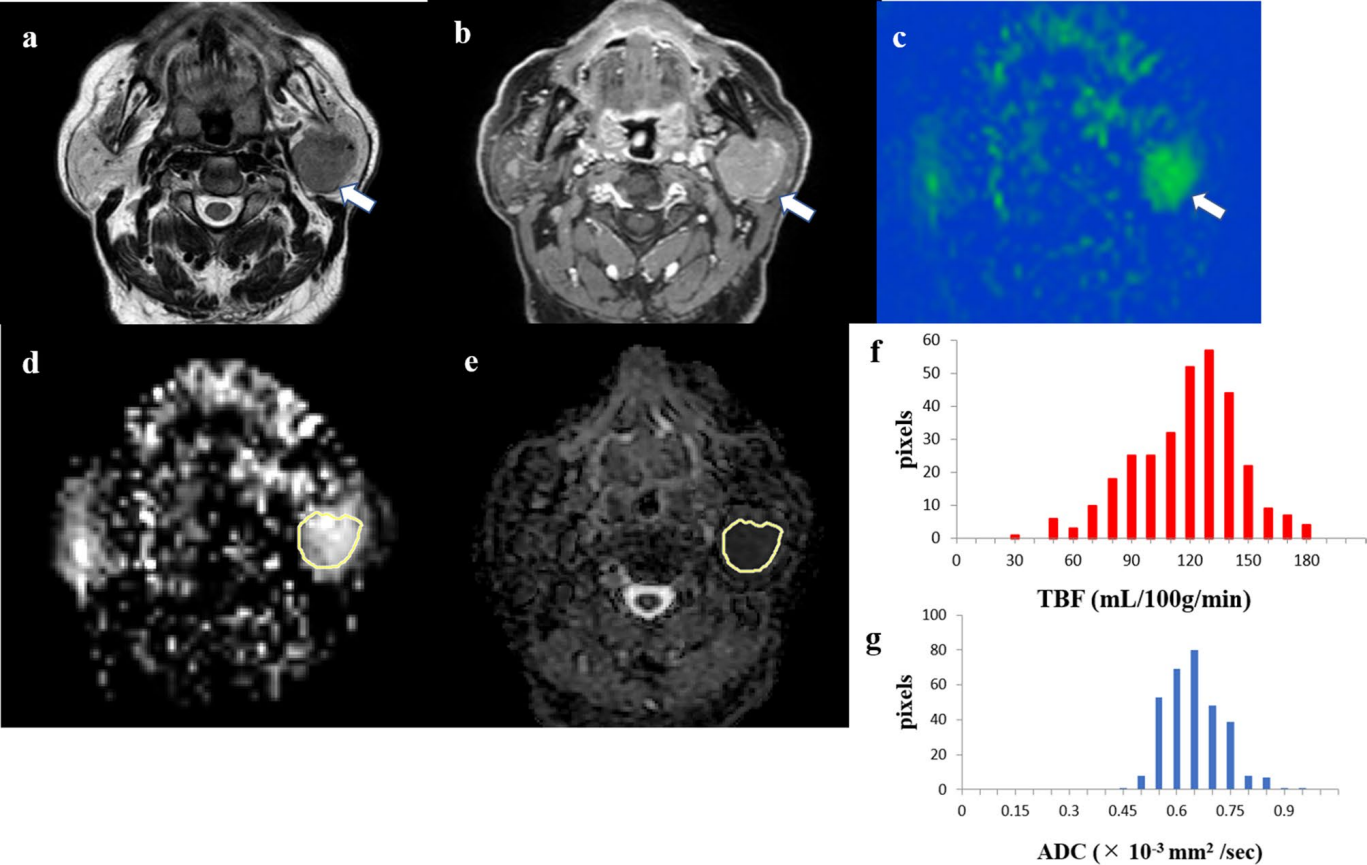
An 83-year-old male patient with a Warthin’s tumor in the left parotid gland. T2-weighted image (a) showing iso signal intensity lesion (*arrow*). Contrast-enhanced 3D-T1-weighted image (b) showing homogeneous contrast enhancement (*arrow*). Tumor blood flow (TBF) color map (c) showing high TBF (*arrow*). The region of interest (ROI) was manually drawn on the apparent diffusion coefficient (ADC) map of the software (e, *yellow*), and the ROI was copied from the ADC map to the TBF map of the software (d, *yellow*). TBF histogram (f) and ADC histogram (g) are presented. The 50th percentile of the TBF value was 117.90 mL/100 g/min, whereas the 10th percentile of ADC value was 0.53 × 10^−3^ mm^2^/s.
